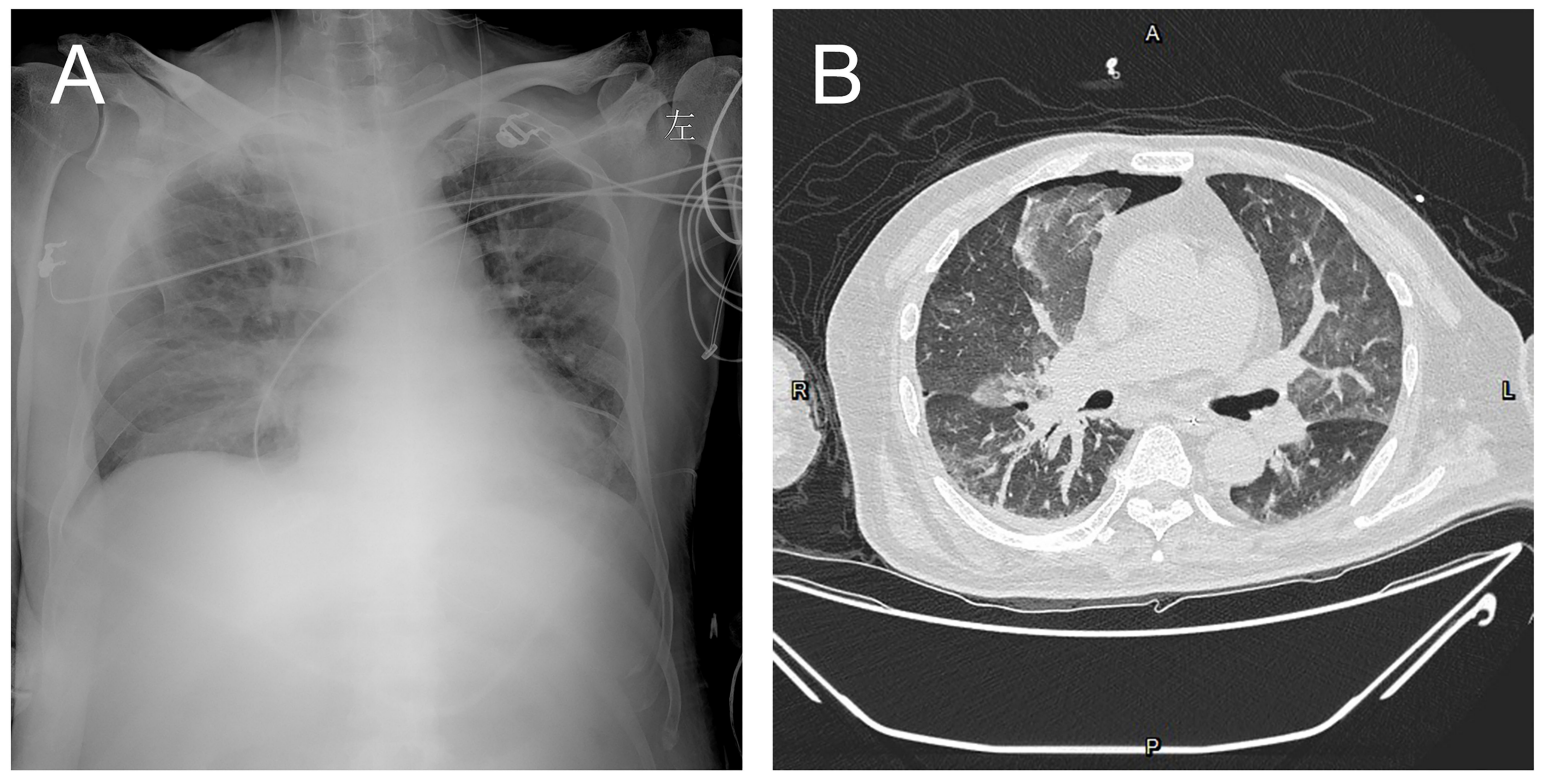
The patients' chest X-ray on July 1st (A) and CT on July 16th (B).
